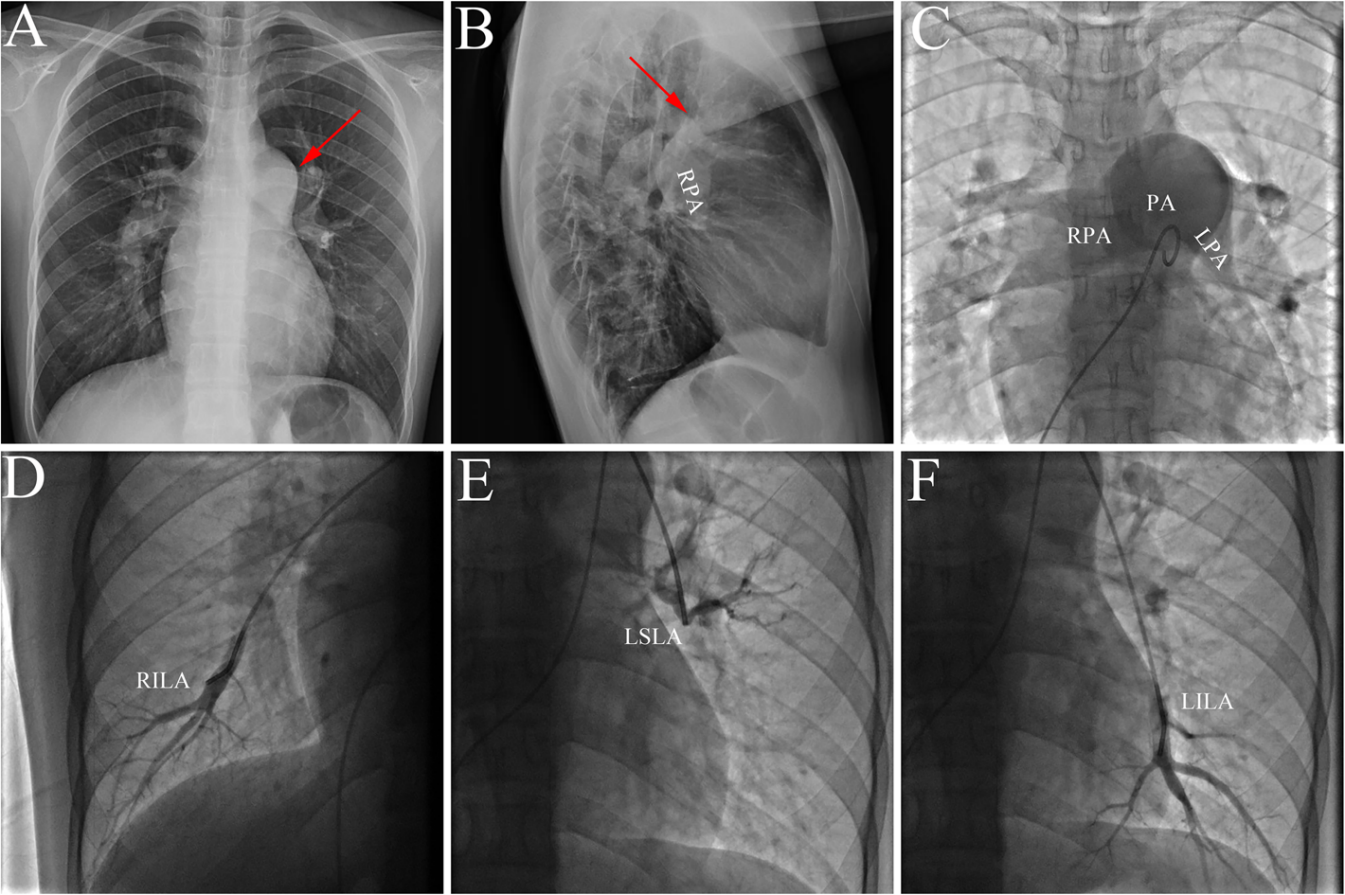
The representative image of PAH-ASD. a and b: Chest X-ray showed cardiomegaly and a bulging pulmonary artery at the upper left cardiac border; c, d, e and f: Pulmonary arteriography showed reduced pulmonary capillary filling. PAH: pulmonary arterial hypertension; ASD: atrial septal defect; PA: pulmonary artery; RPA: right pulmonary artery; LPA: left pulmonary artery; RILA: right inferior lobe artery; LSLA: left superior lobe artery; LILA: left inferior lobe artery
